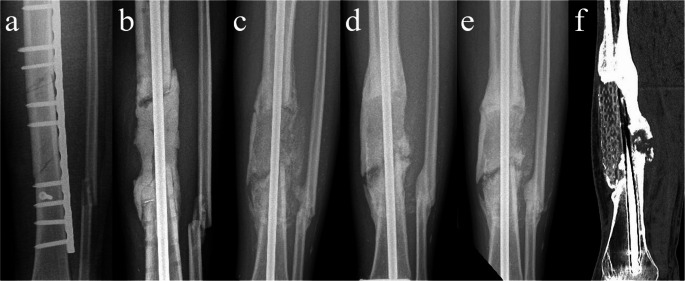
Radiological follow-up of one of the patients (pt.5). Images are in chronological order: a – Preoperative image of the initial non-union for which the patient was referred, b – Cement in situ after first stage IMT, c – After second stage procedure and implantation of the scaffold, d – Six months postoperatively with ongoing bone formation proximal and medial, e – One and a half year after IMT shows stunted bone formation. The CT-scan (f) made after the last radiograph at one and a half year shows a persisting non-union with an inert scaffold
